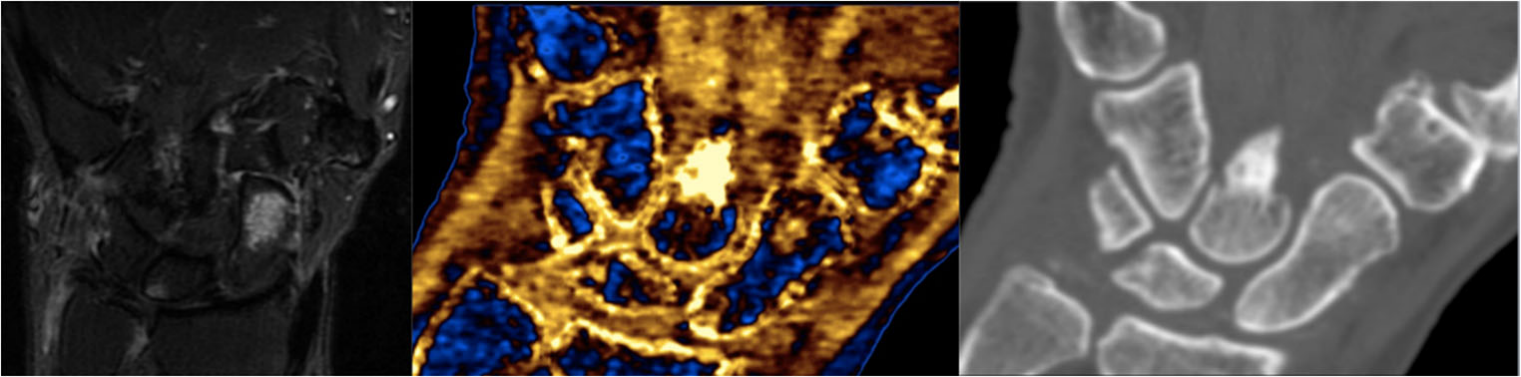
Focal and central acute scaphoid oedema. Both STIR sequence of the MRI (left) and DECT with calcium/water filter (middle) showed focal and central acute scaphoid oedema at the distal pole and waist of the scaphoid. The oedema is highlighted in gold on the DECT with calcium/water filter image. The CT component (right) did not show any fracture
